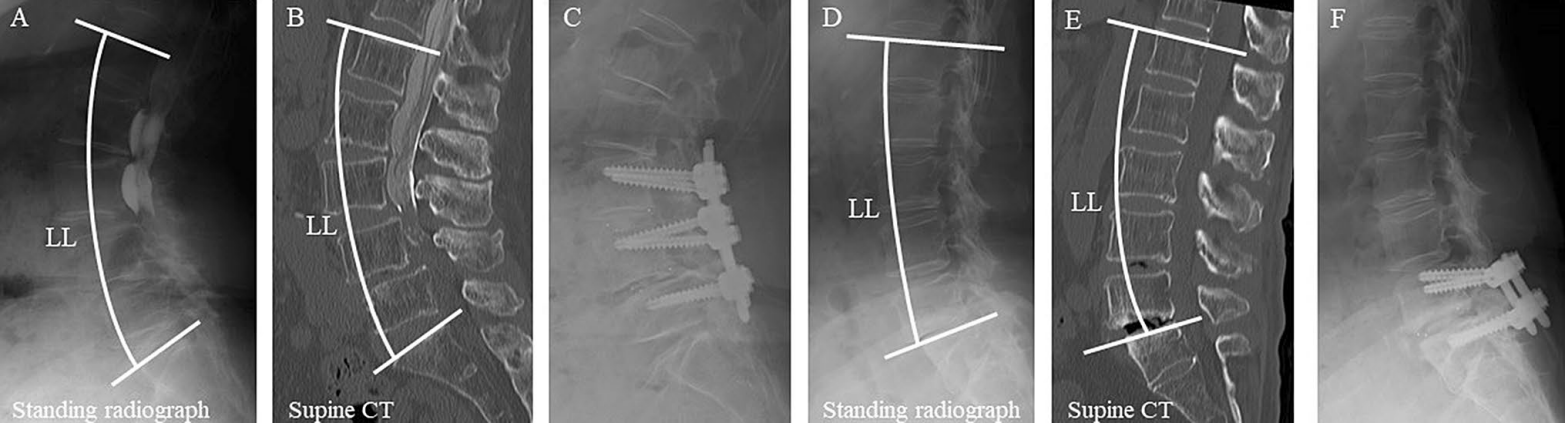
Pre-operative lateral radiographs obtained in the standing position (A,D), computed tomography (CT) images obtained in the supine position (B,E), and postoperative lateral radiographs taken after short-segment transforaminal lumbar interbody fusion (C,F) of DiLL (−) patient (A–C, DiLL < 0°) and DiLL (+) patient (D–F, DiLL ≥ 0°). In DiLL (−) patient, the lumbar lordosis (LL) is greater in the standing radiograph (A) than in the supine CT (B), while the LL is smaller in the standing radiograph (D) than in the supine CT (E). *DiLL* difference in lumbar lordosis between the standing and supine positions.
